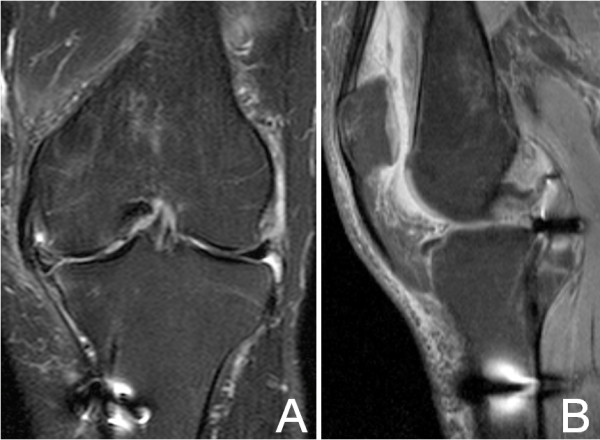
MRI in T1 sequences 10 months after CMI implantation. In coronal plane (6A) the meniscal complex remains in contact with medial collateral ligament and triangular meniscal shape is observed. In the sagittal plane (6B) posterior graft lies completely over the posterior tibial plateau.
